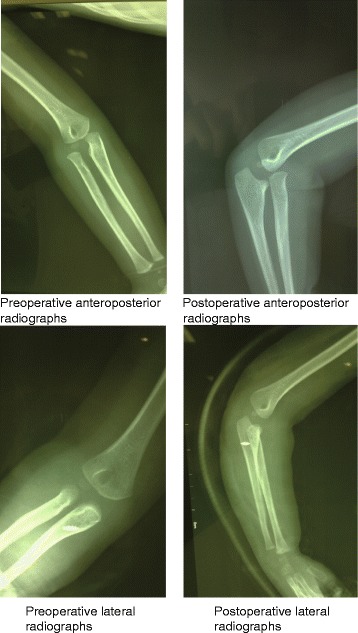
X-ray film of a 10-year-old boy (case 9) with left radial head dislocation before and after treatment with reconstruction of annular ligament by suture anchor. a X-ray in the right view of the elbow before surgery; b X-ray in the lateral view of the elbow before surgery; c X-ray in the right view of elbow after surgery; d X-ray in the lateral view of the elbow after surgery
